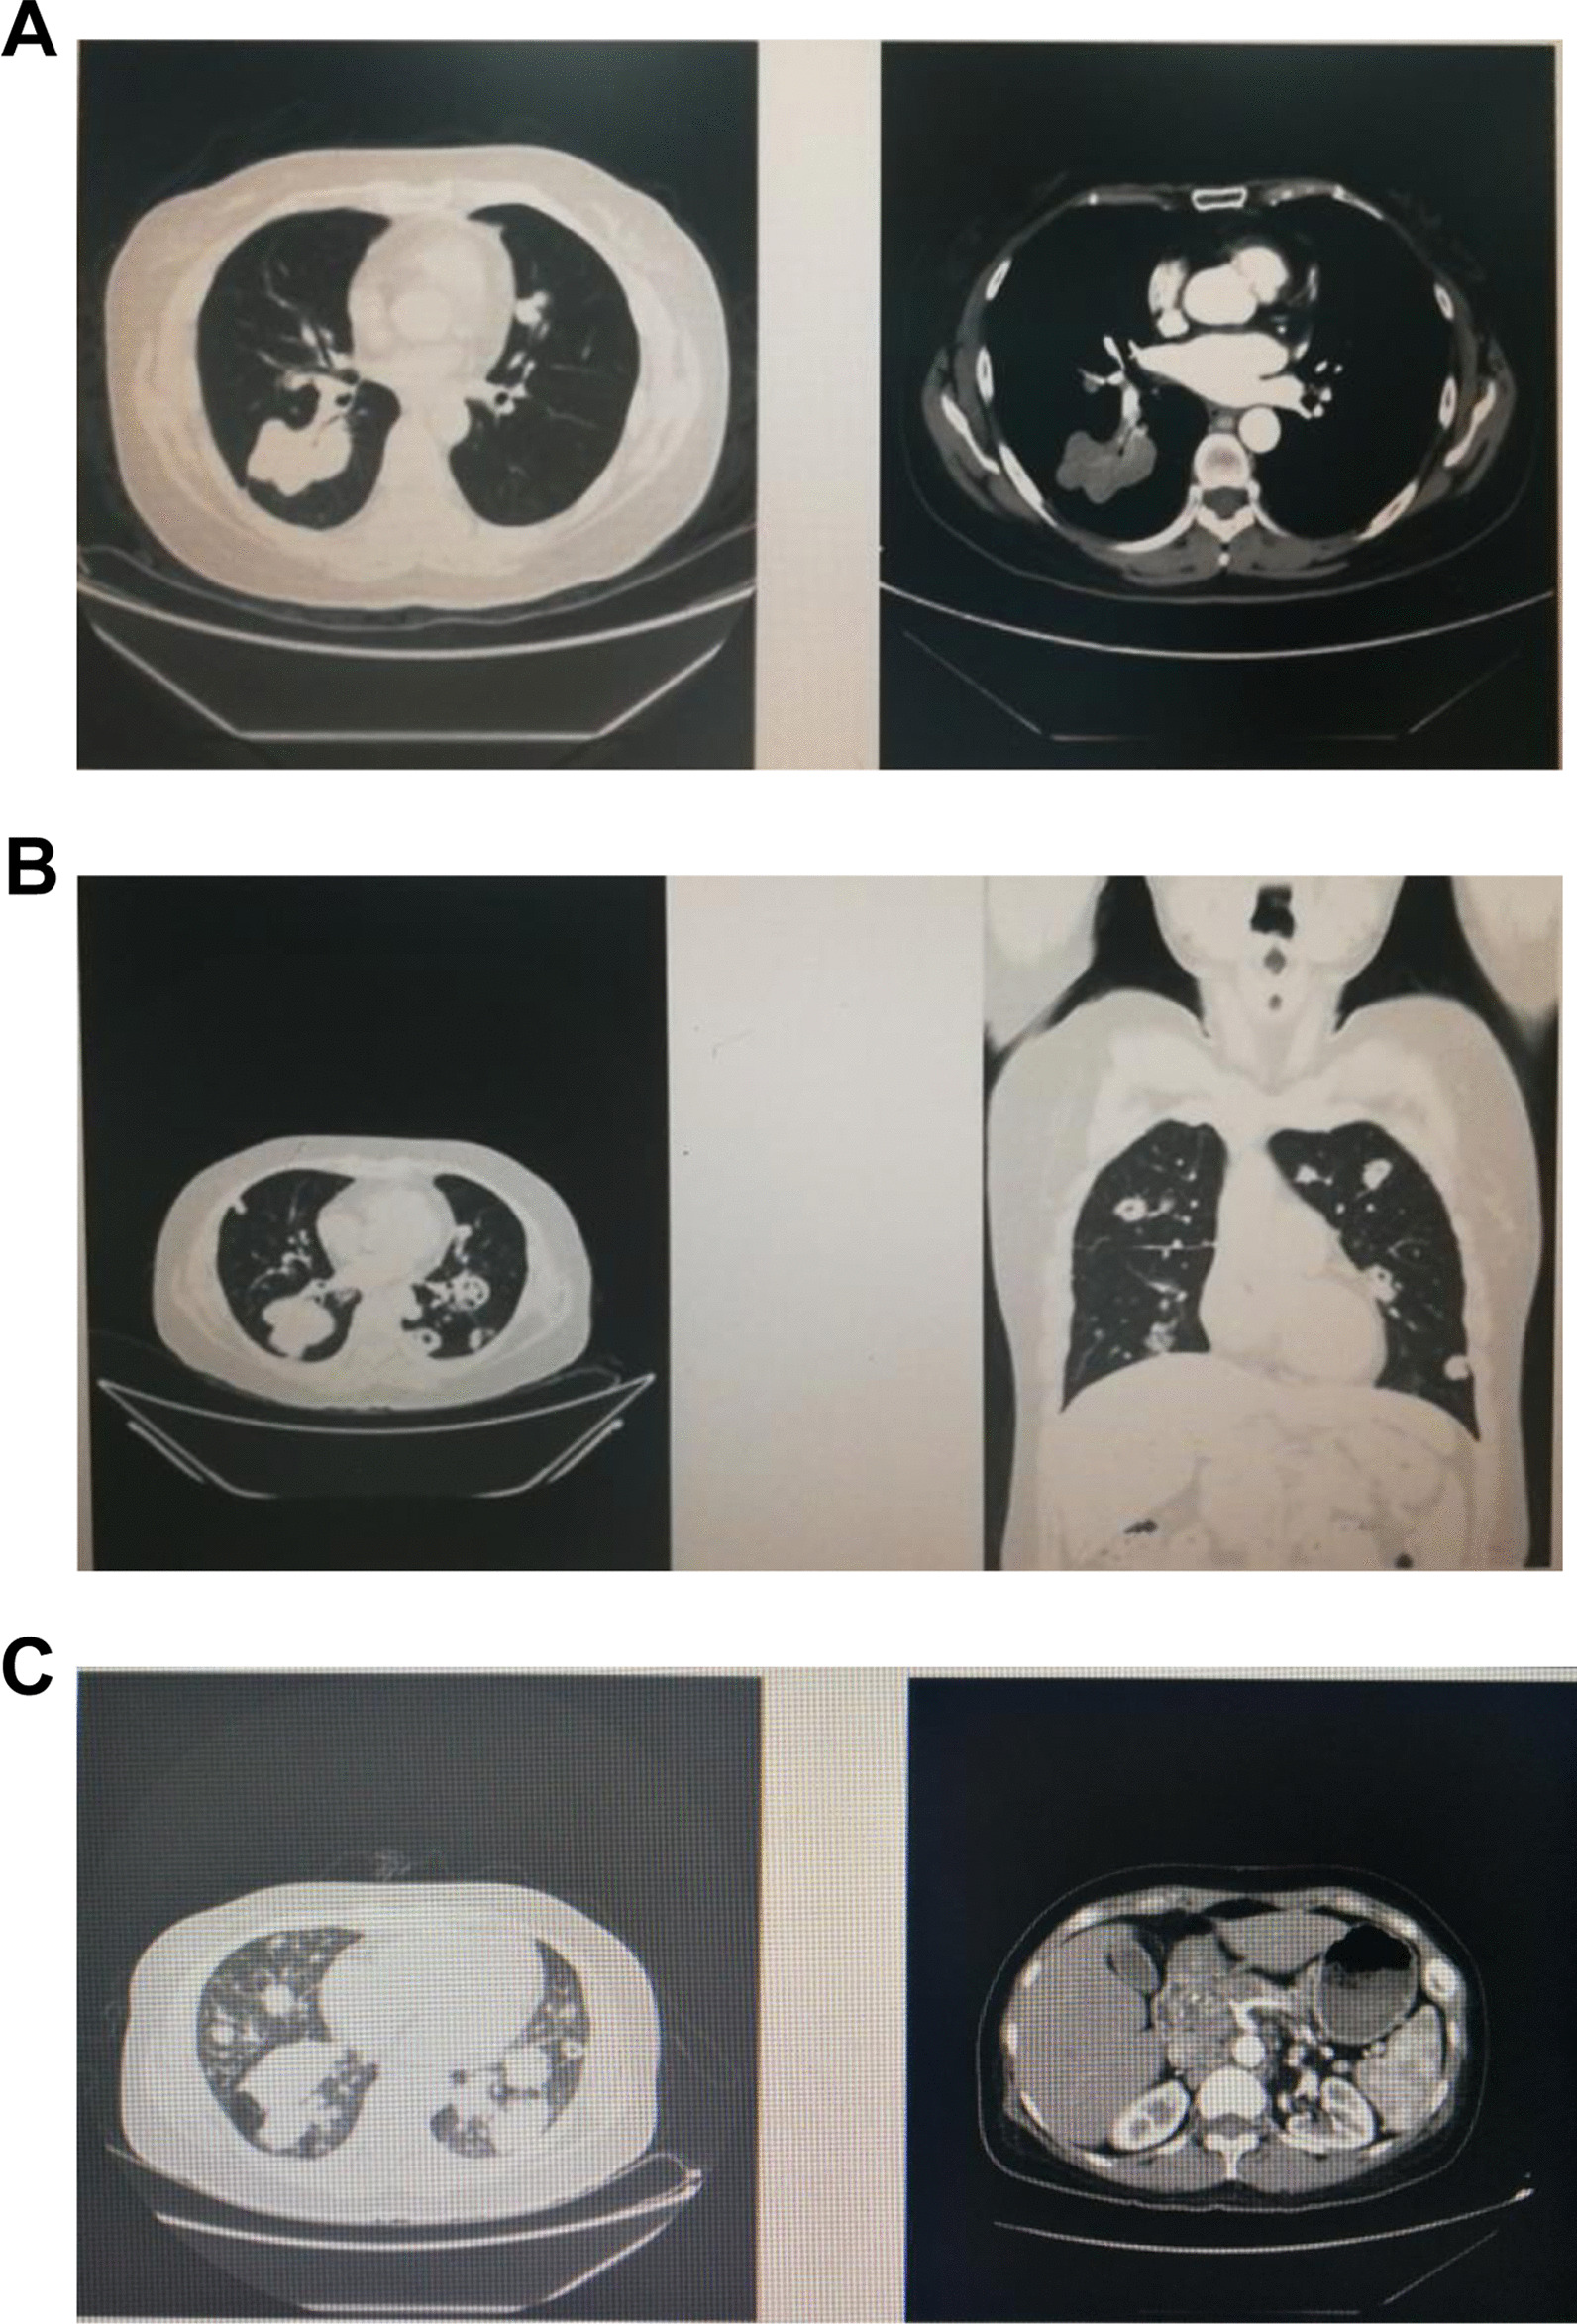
A CT results (December 20th, 2019) indicated that some bilateral lung nodules were slightly reduced, and some nodules showed small cavities, suggesting progressive disease. B CT results (May 10th, 2019) suggested increased and enlarged bilateral lung nodules and some cavities appeared. C CT results (September 14th, 2020) showed increased and enlarged bilateral lung nodules, as well as enhanced cervical and thoracic lesions
